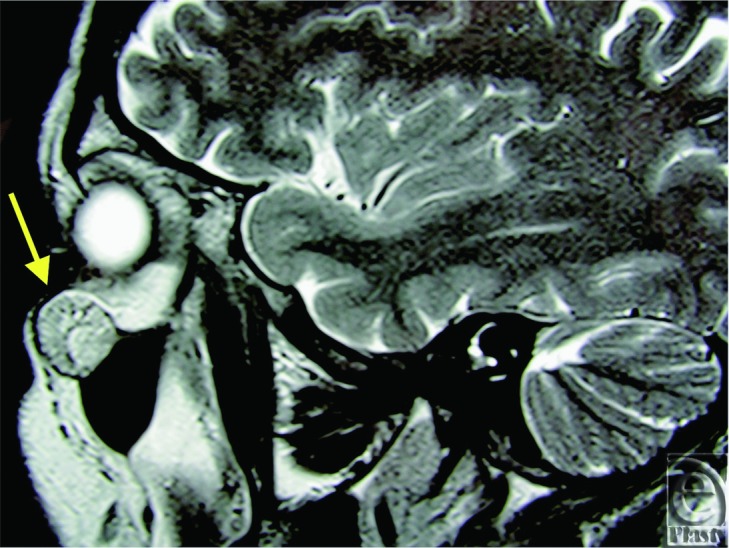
Sagittal MRI image of the infraorbital lesion.
